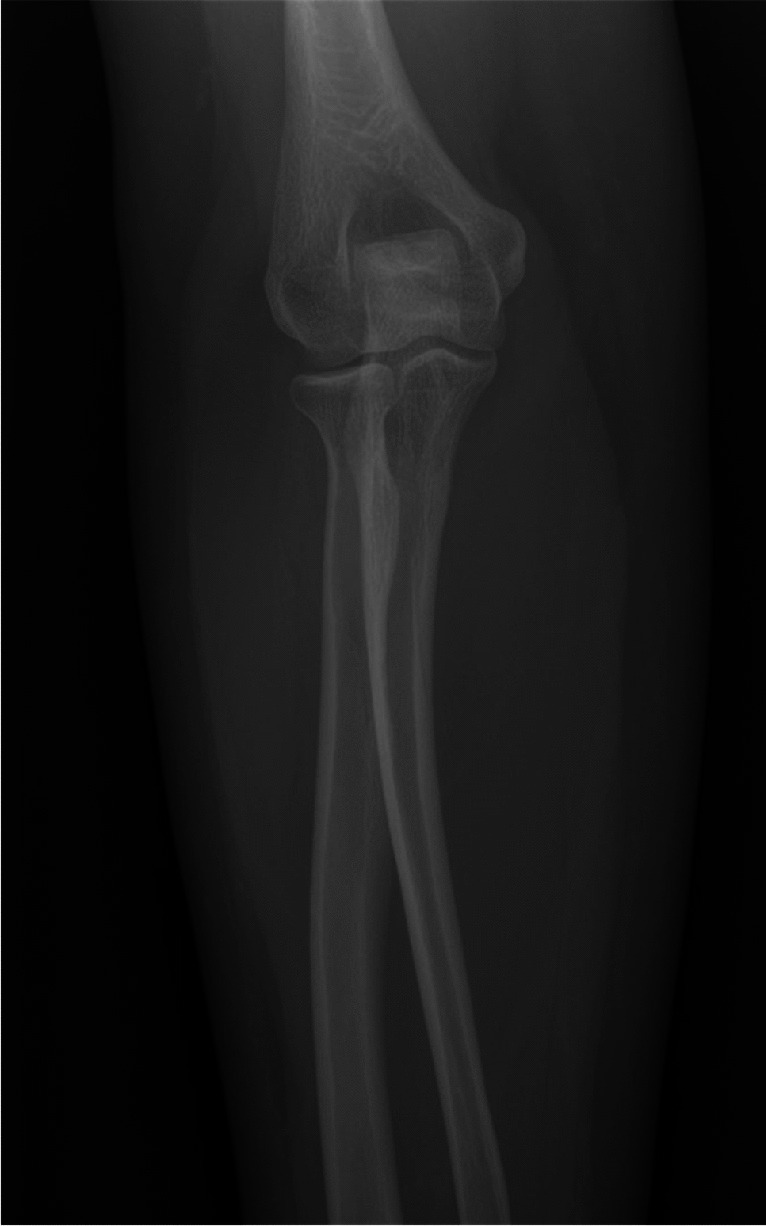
AP radiograph of the right forearm demonstrates no radiographic osseous or soft tissue abnormality
